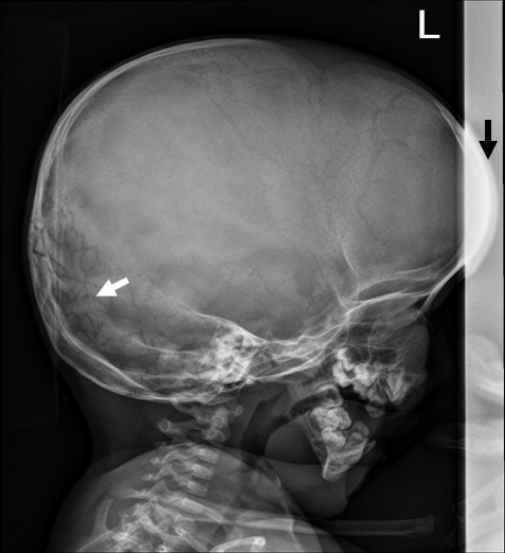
Lateral skull radiograph showed profound frontal bossing (black arrow), thin calvarium, absence of the mandibular angle (temporomandibular joint anomaly with subsequent malocclusion of the teeth), defective ossification of the anterior fontanelle, abundant wormian bones (white arrow) and J-shaped cella turcica.
